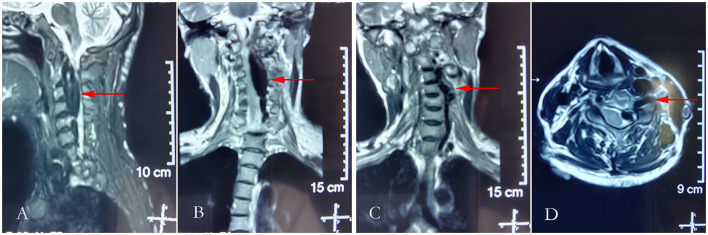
Cranial T2-weighted magnetic resonance imaging. (A) Sagittal plane of the neck; (B,C) the coronal plane of the neck; and (D) horizontal plane of the neck.
